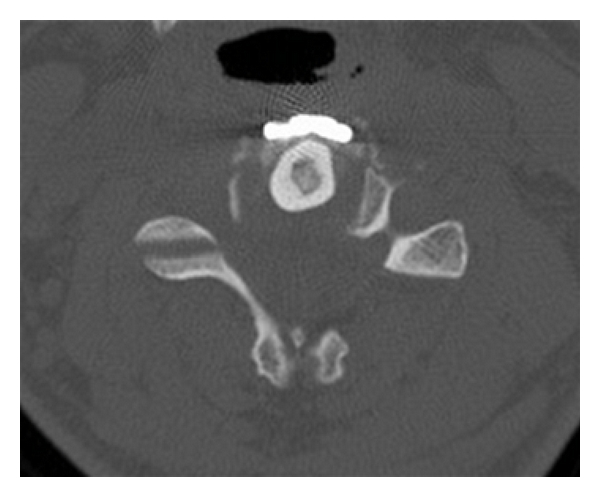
Axial CT scan demonstrating fibula strut graft placed in central corpectomy trough.
